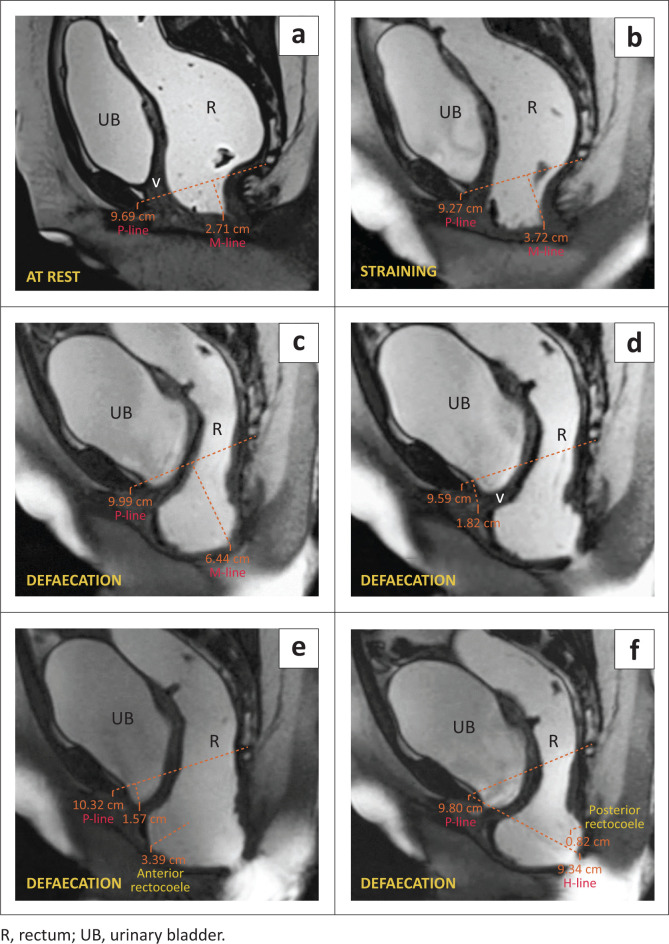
(a–f) MRI defaecogram: Obstructed defaecation with Grade 3 anorectal descent, anterior and posterior rectocoele. (a) At rest, (b) on straining, (c) during defaecation, shows progressive Grade 1, 2, and 3 anorectal descent. (d) During defaecation, mild vaginal vault prolapse was seen. (e) Defaecation phase demonstrates a mild cystocoele and medium anterior rectocoele. (f) The defaecating sequence shows a small posterior rectocoele with an increased H-line representing hiatal widening.
